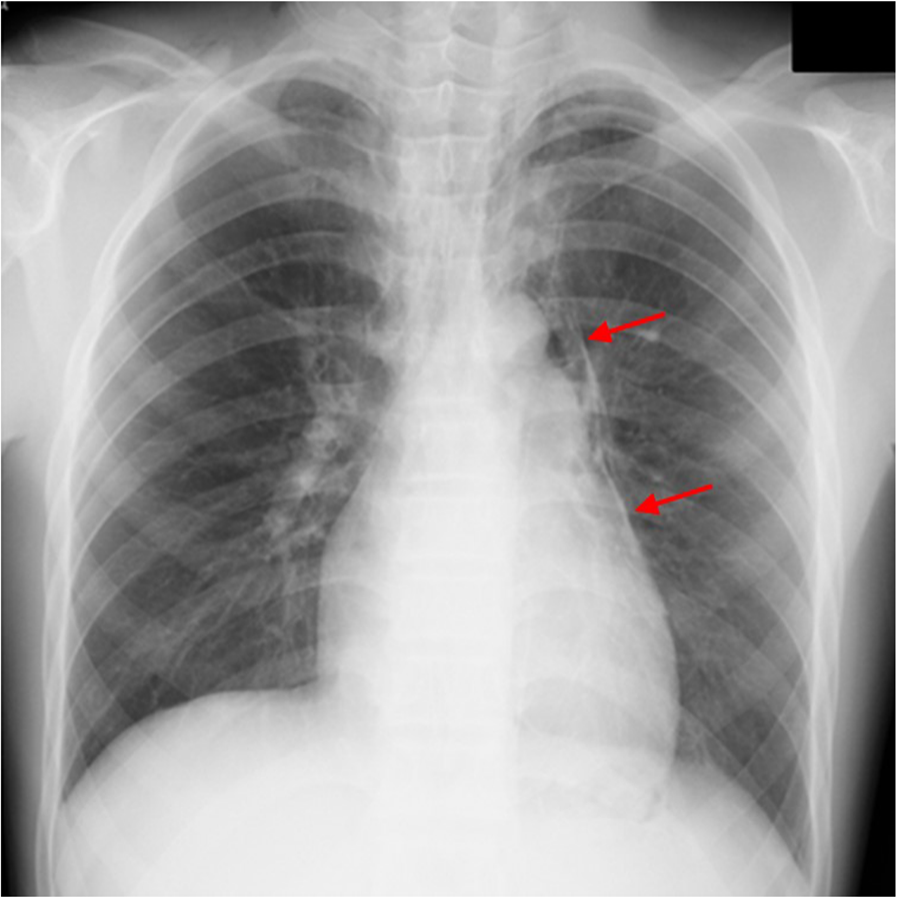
A chest radiograph of the mediastinal pleura
